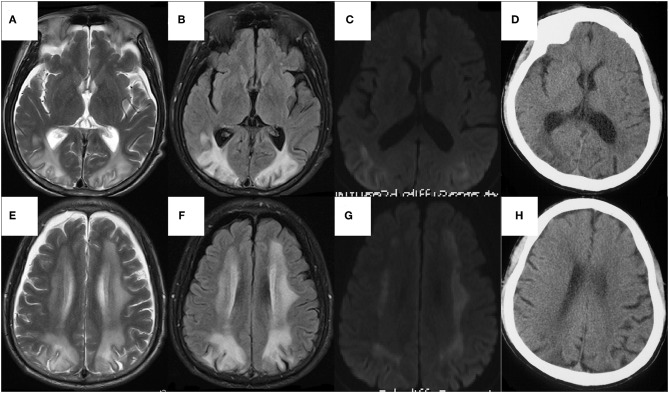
The axial sequences on head MRI of the patient (A–F) and The head CT of the sick brother (G,H). (A–F) Bilateral symmetric diffuse white matter hyperintensity, predominantly involving the frontal, parietal, occipital, and periventricular regions on T2WI (A,B) and FLAIR (C,D), with restriction in peripheral areas of lesions on DWI (E,F), whereas normal on ADC map. The U-fibers of bilateral occipital lobe and the white matter of paracortical, subcortical were involved but corpus callosum and internal capsule were relatively reserved. There was atrophy in temporal and frontal lobes, especially significant in frontal pole. (G,H) The low-density lesions around bilateral ventricles mainly involving white matter around the posterior horn of lateral ventricles, with atrophy of temporal and frontal lobes especially in frontal pole.
